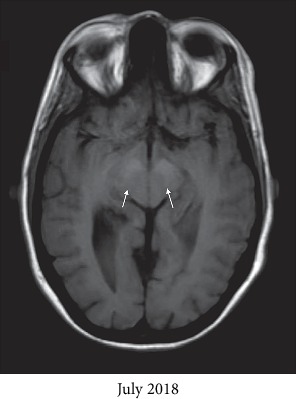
Axial T1-weighted MRI brain from July 2018 showing hyperintensities in the substantia nigra, as evidenced by the two white arrows.
